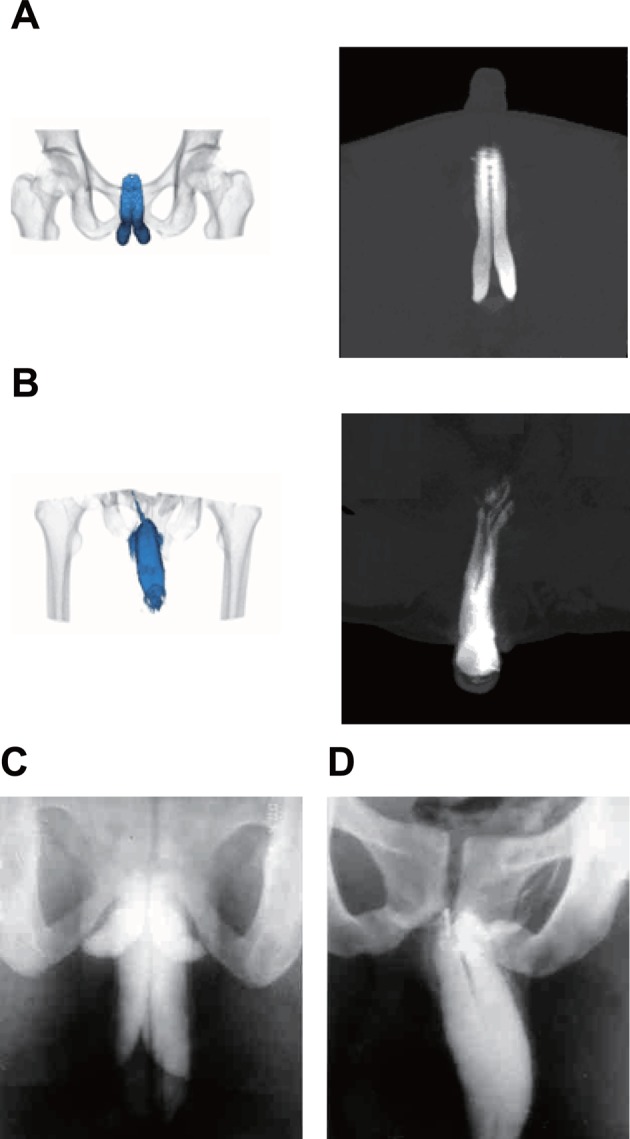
Image of corpora cavernosum detected by the cavernosography with 320-row DVCT compared with an image taken by conventional cavernosography (A) Cavernosography with 320-row DVCT image of regular cavernosa by; (B) cavernosography with 320-row DVCT image of cavernosal venous leakage by; (C) conventional cavernosonography image taken of the regular cavernosa by; (D) conventional cavernosa image of the cavernosal venous leakage by DVCT.
